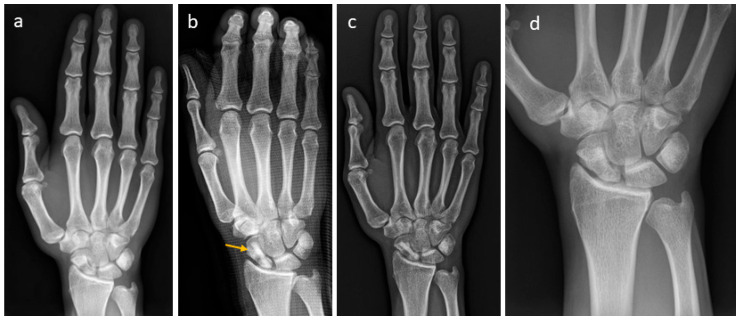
A patient with a non-union of the scaphoid. (a): presurgical X-ray; (b): the patient was treated with one Shark Screw^®^ (orange arrow); (c): union was obtained after eight weeks. (d): After 35 months, the Shark Screw^®^ is totally remodelled into host bone.
